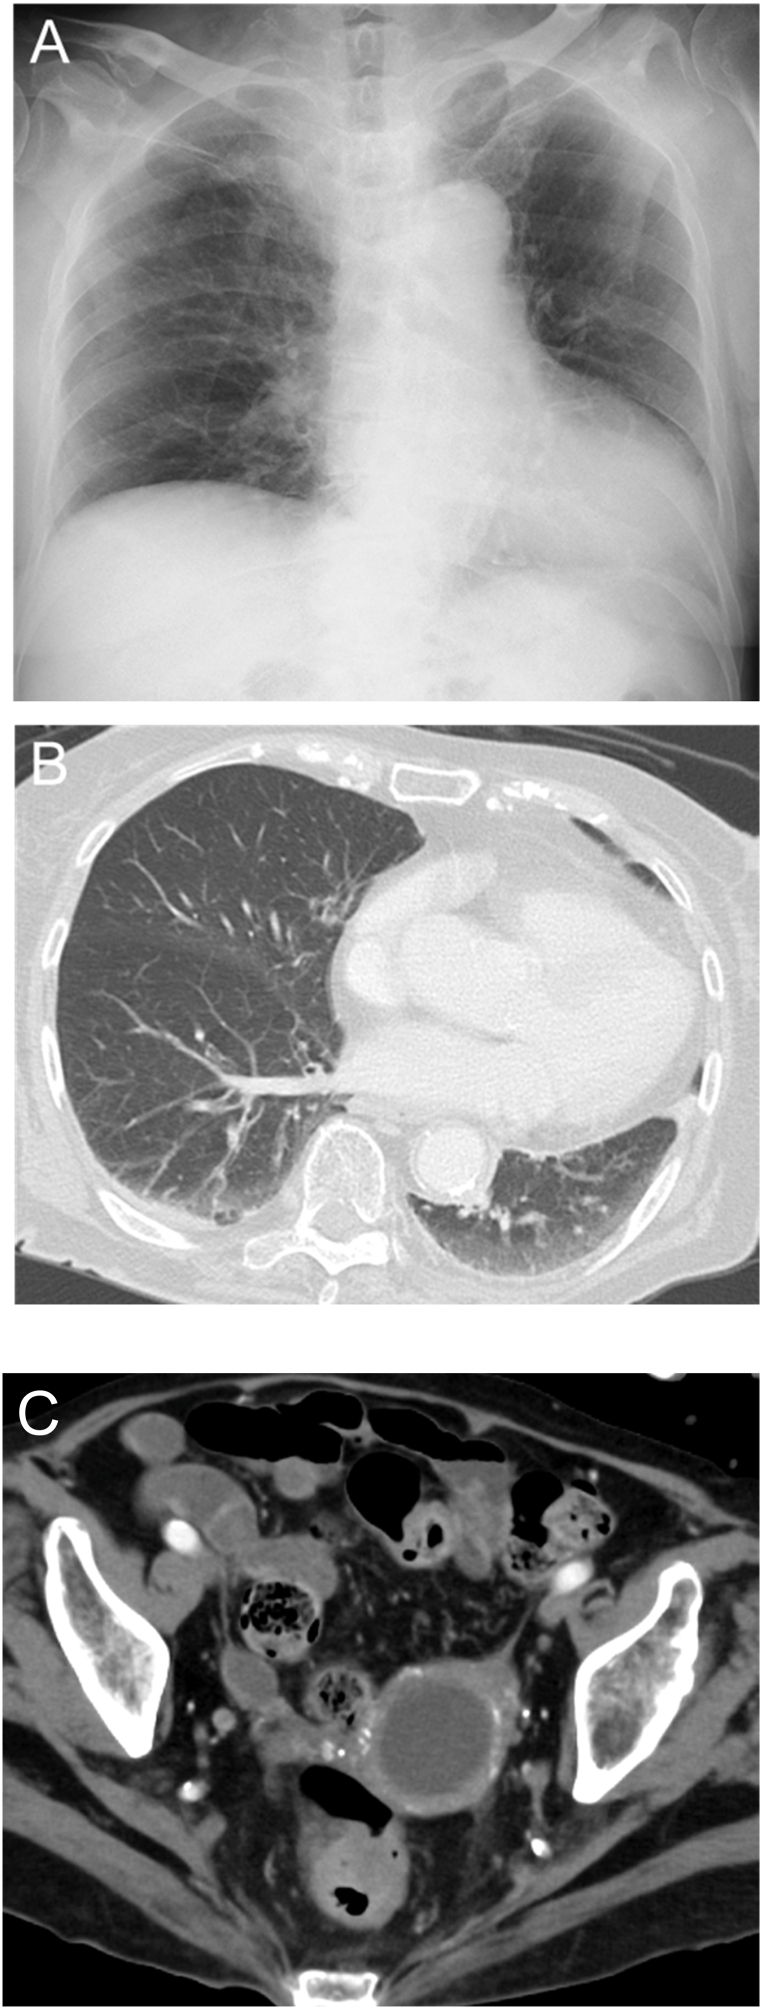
CT findings at one month before admission. A. Chest CT demonstrated no evident abnormalities in the lung fields. B. Abdominal and pelvic CT demonstrated the uterine pyometra.
